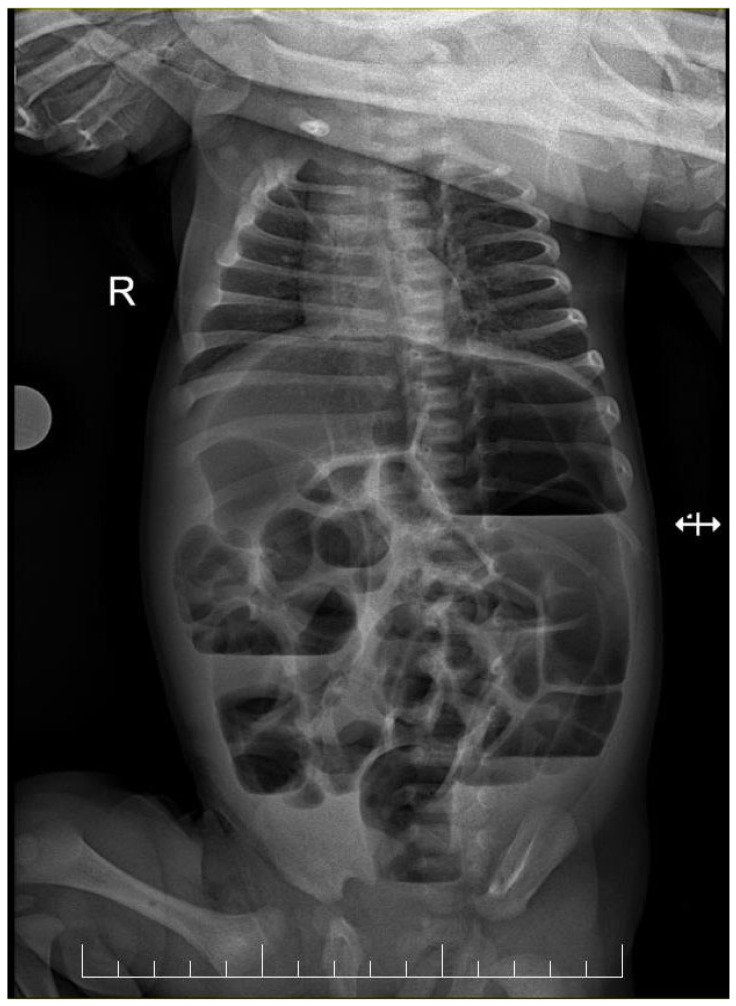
Plain abdominal radiography showing multiple air–fluid levels and significant gaseous bowel distension, suggestive of intestinal obstruction in an 18-day-old neonate. (R: right side; double-headed arrow: horizontal X-ray beam).
